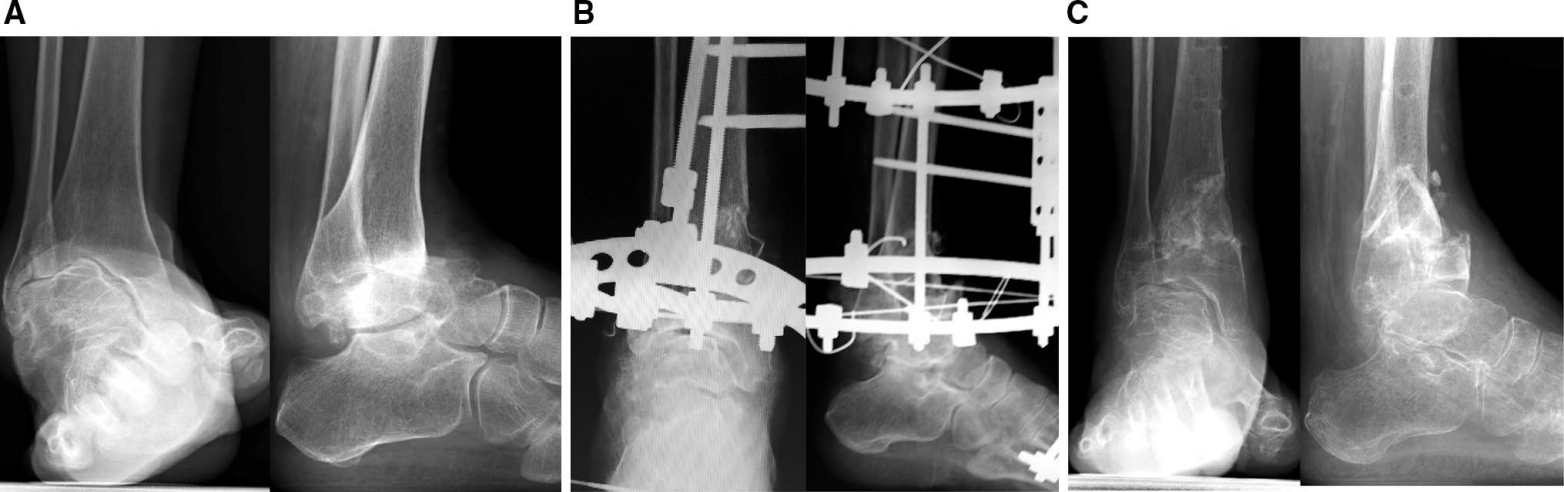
A 70-year-old woman with stage 4 varus ankle osteoarthritis of her right ankle underwent a DTOO. The weight-bearing radiograph before surgery shows severe varus deformity and joint space narrowing (a). During surgery and after the osteotomy, ankle dorsiflexion was reduced to − 10°; the Achilles tendon was elongated (20 mm). Bony spurs present in the fibula and the tibia are removed because these spurs prevented satisfactory restoration of ankle joint congruity. The severe ankle joint instability and subluxation was improved after the DTOO. Fixation was by Ilizarov external fixator (b). Weight-bearing radiographs 2 years after the DTOO show the good restoration of the ankle mortise, with the plantar surface of the hindfoot perpendicular to the tibial axis (c). The range of motion was maintained (dorsiflexion 5°—plantar flexion 25°). The patient reported absence of symptoms and a return to activities of daily living without pain
